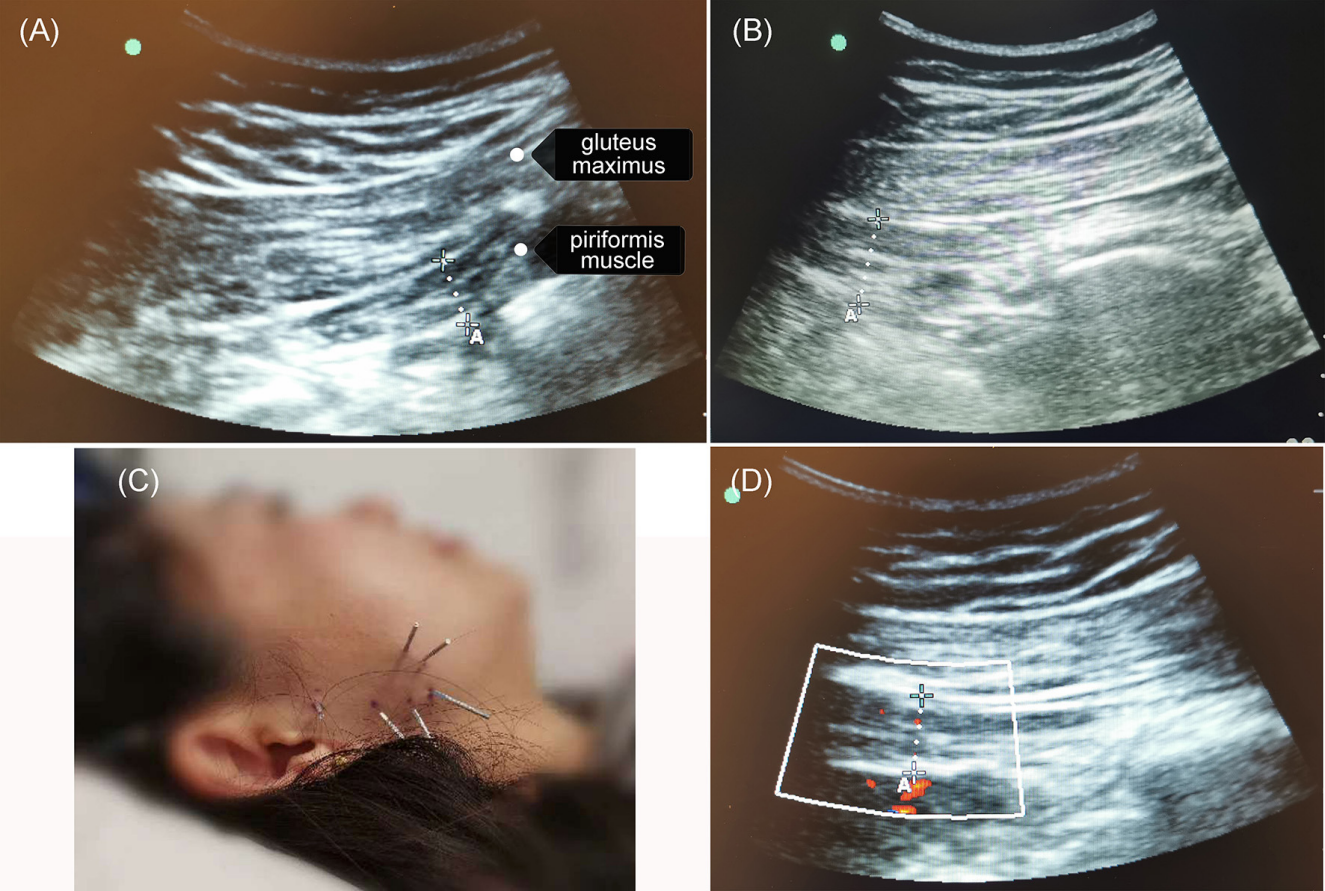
The cheek acupuncture reduced the thickness of the piriformis muscle. On day 7 after the first acupuncture session, an ultrasound was performed to measure the thickness of the piriformis muscle of the hip before and after the second session of treatment. (A) The piriformis muscle in the left hip (healthy) was 1.18 cm. (B) Before the second session of acupuncture, the thickness of the piriformis muscle in the right hip (injured) was 2.05 cm. (C) The selected acupoints for acupuncture treatment. (D) The piriformis muscle in the right hip (injured) decreased to 1.31 cm post-second treatment.
